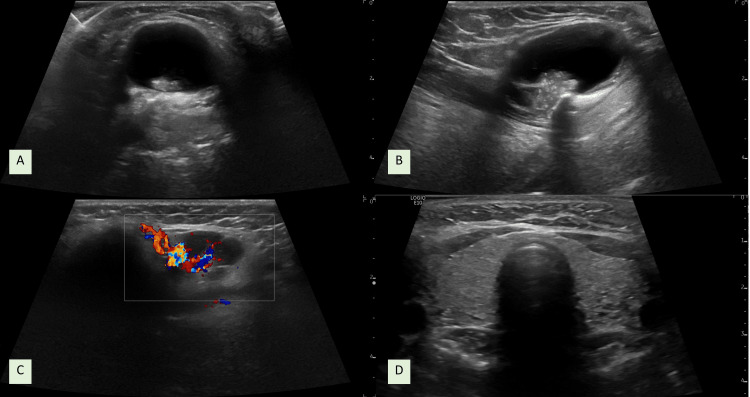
First cervical ultrasonography A and B: Thyroglossal duct cyst transversal and longitudinal views, C: Thyroglossal duct cyst showing solid component with positive Doppler sign, D: Thyroid gland transversal view
